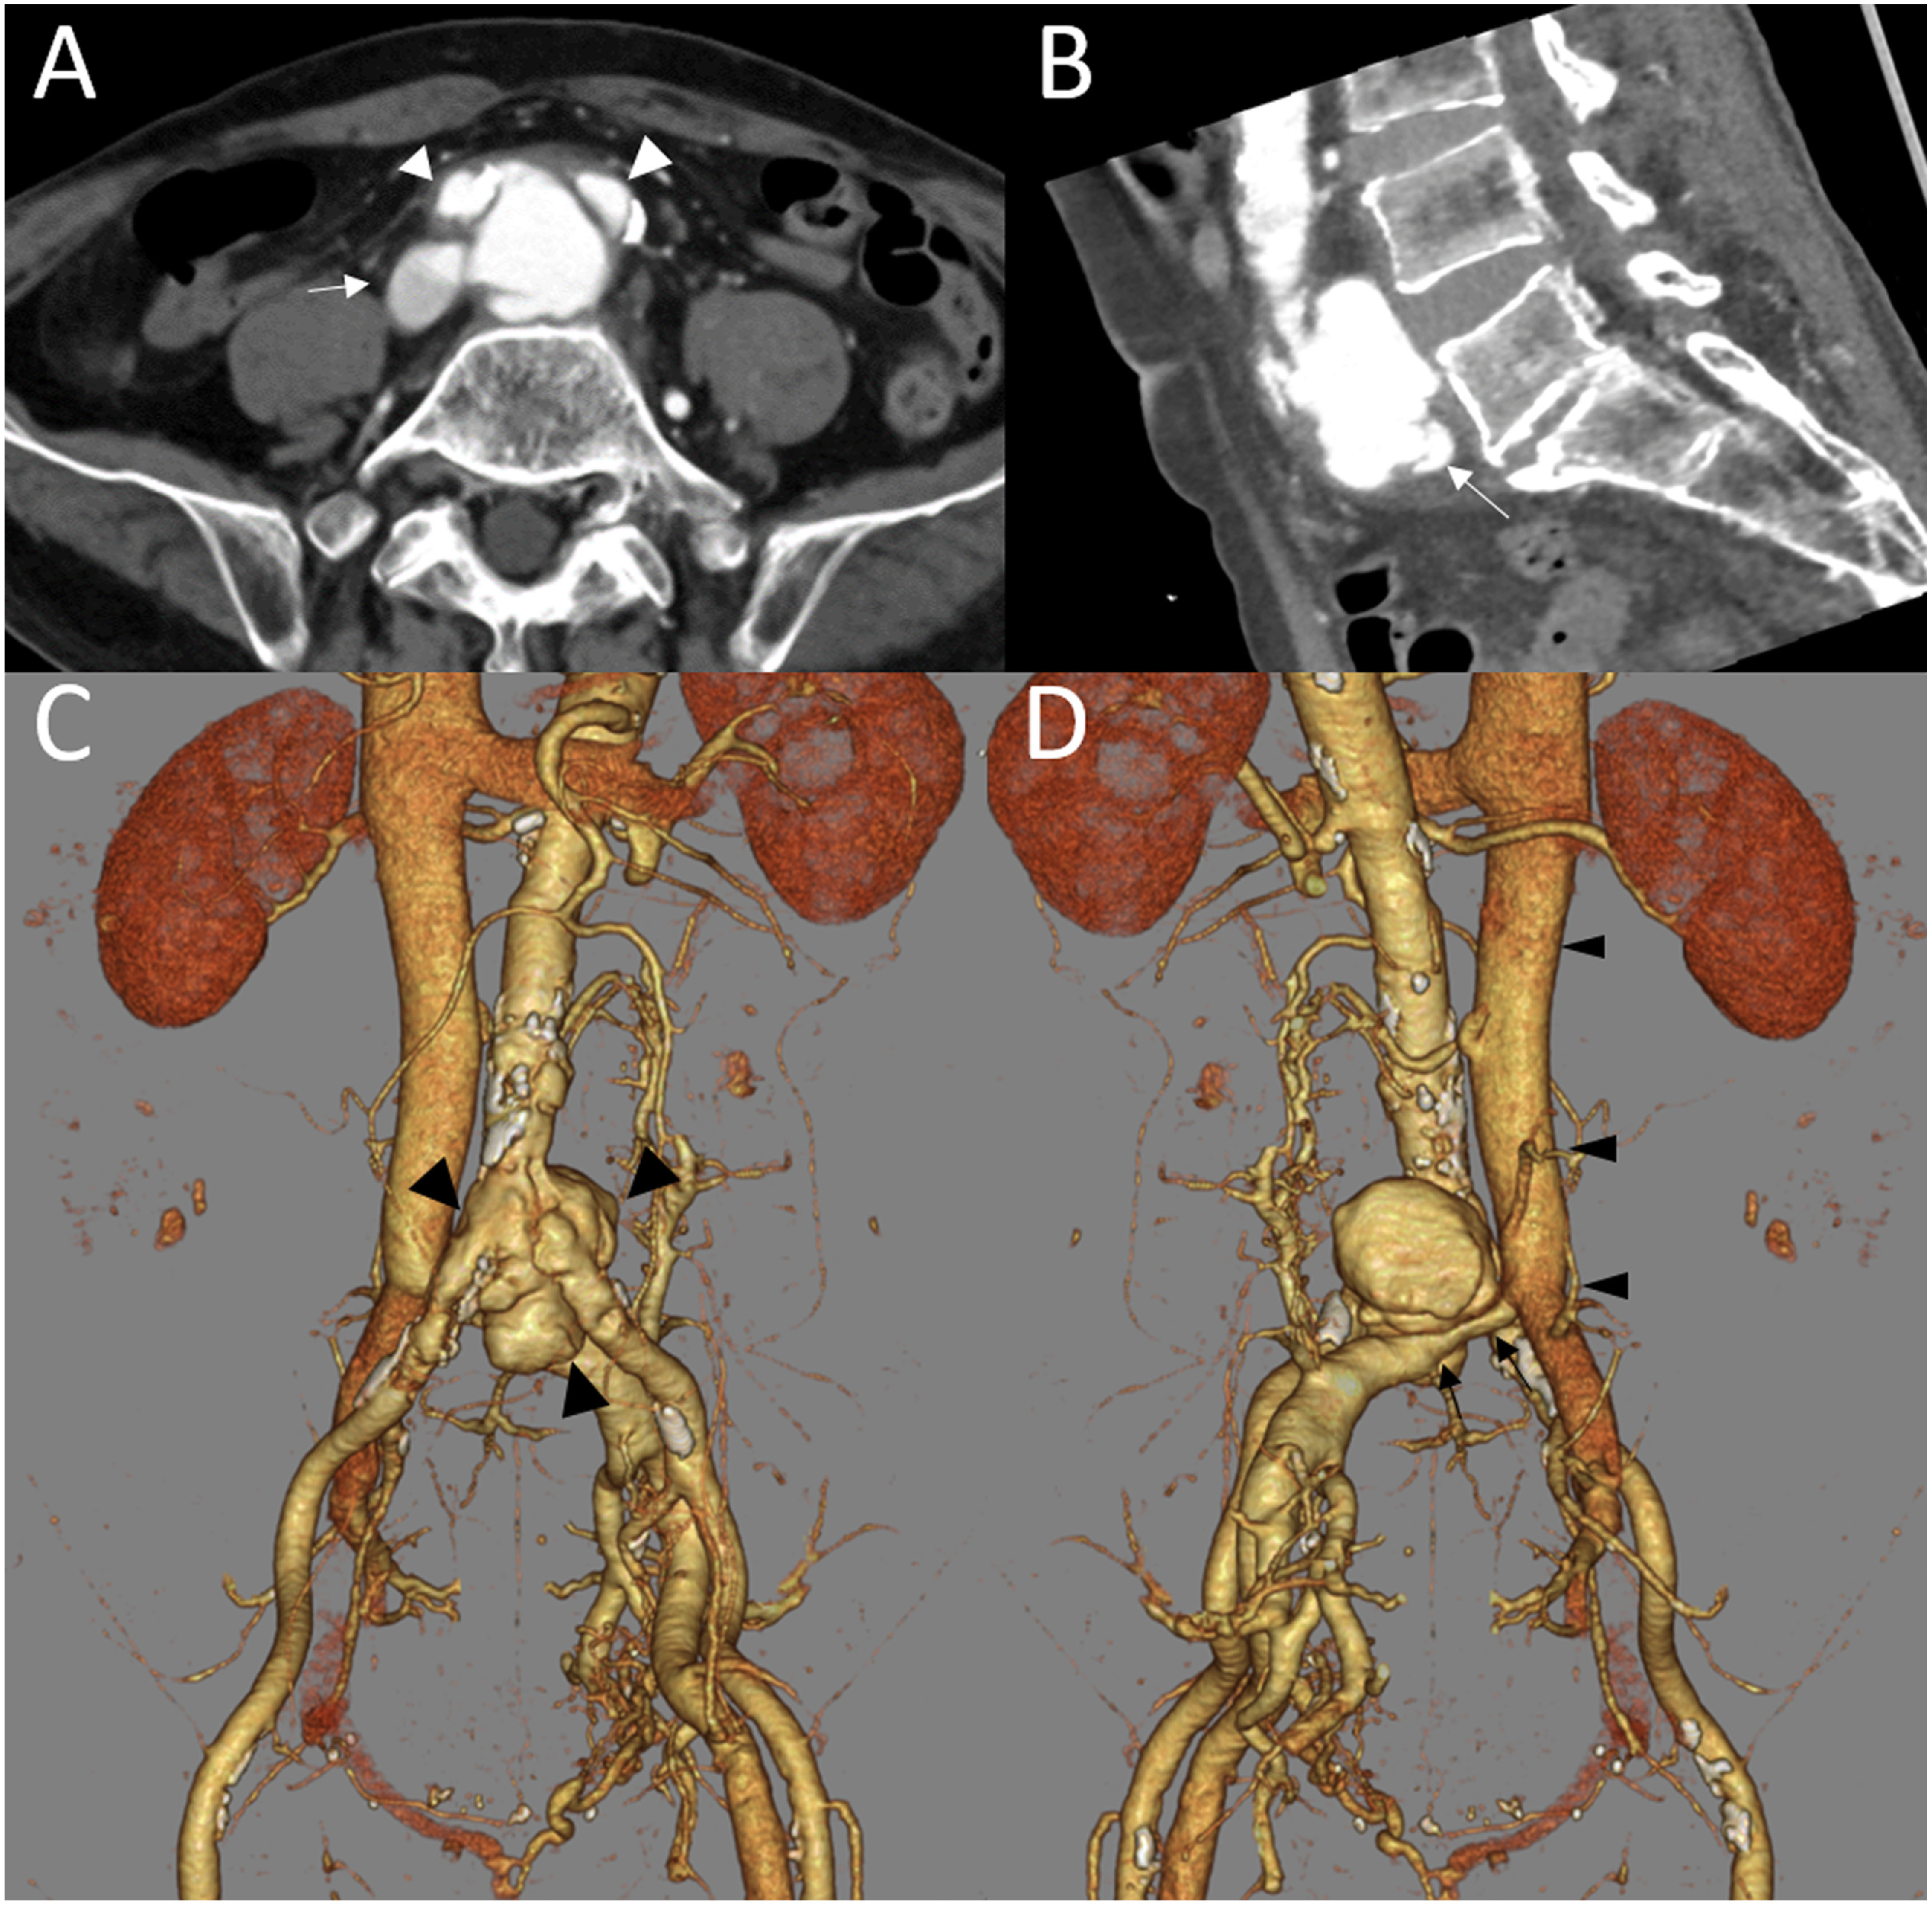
Fig. 1 Preoperative computed tomography (CT) findings. (A) Axial view of the abdominal aortic aneurysm (AAA). The AAA has a diameter of 41 mm and an irregular margin. The arrowheads indicate bilateral common iliac arteries, and the arrow indicates the left common iliac vein, which was enhanced in the early phase. (B) Sagittal view of the AAA extracted from the four-dimensional CT findings. The arrow points to the left iliac vein, which is compressed by the aneurysm and has a fistula communicating with the AAA. (C) Three-dimensional CT findings from the ventral aspect. The aneurysm is located at the level of the aortic bifurcation, as indicated by the arrowheads. (D) Three-dimensional CT findings from the dorsal aspect. The left iliac vein and inferior vena cava are enhanced at the same time as the aorta, as indicated by the arrowheads. The left common iliac vein is compressed by the aneurysm, as indicated by the arrows.
